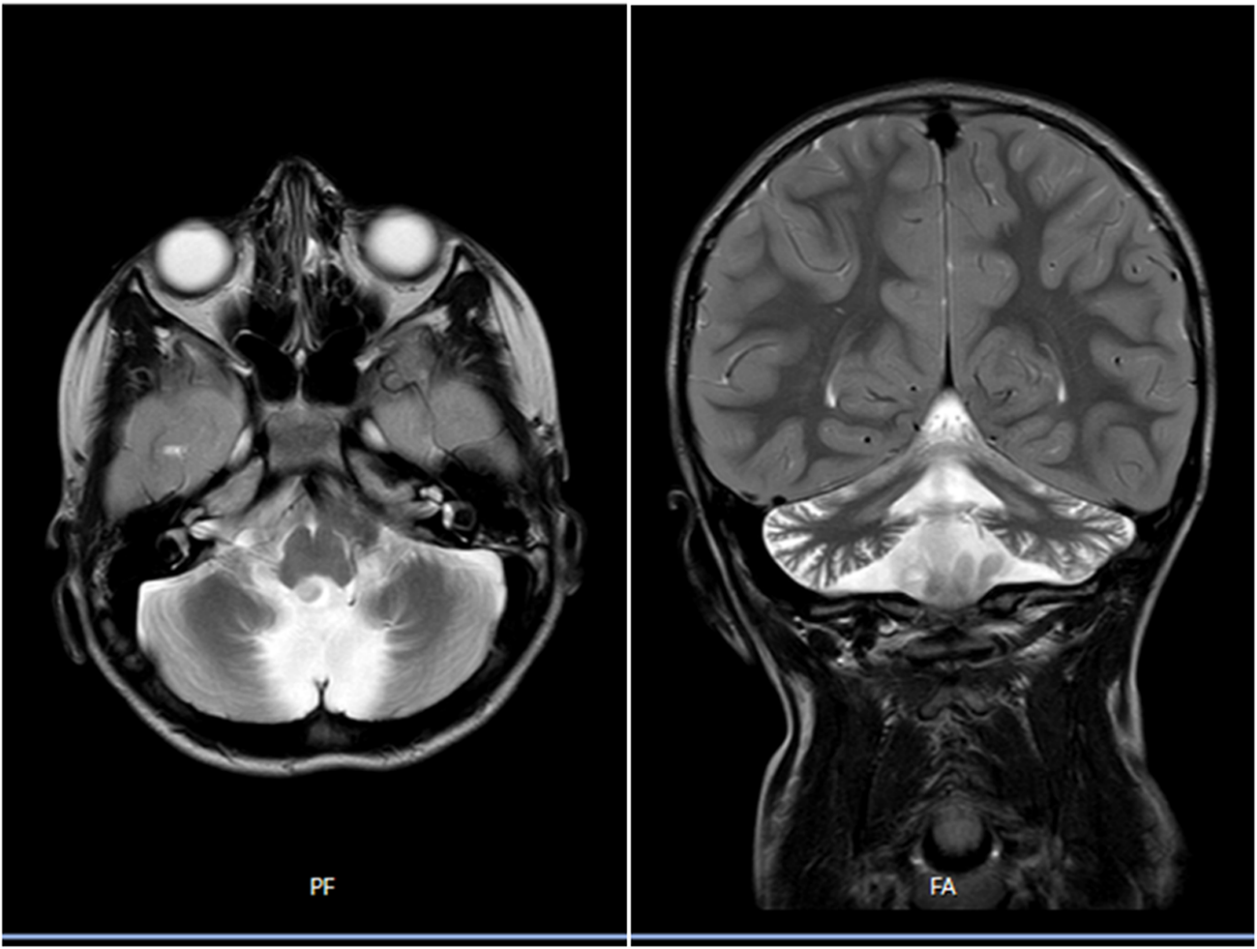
Brain magnetic resonance imaging (MR) sections at 8 years of age with coronal and sagittal midline change showing marked cerebellar atrophy
